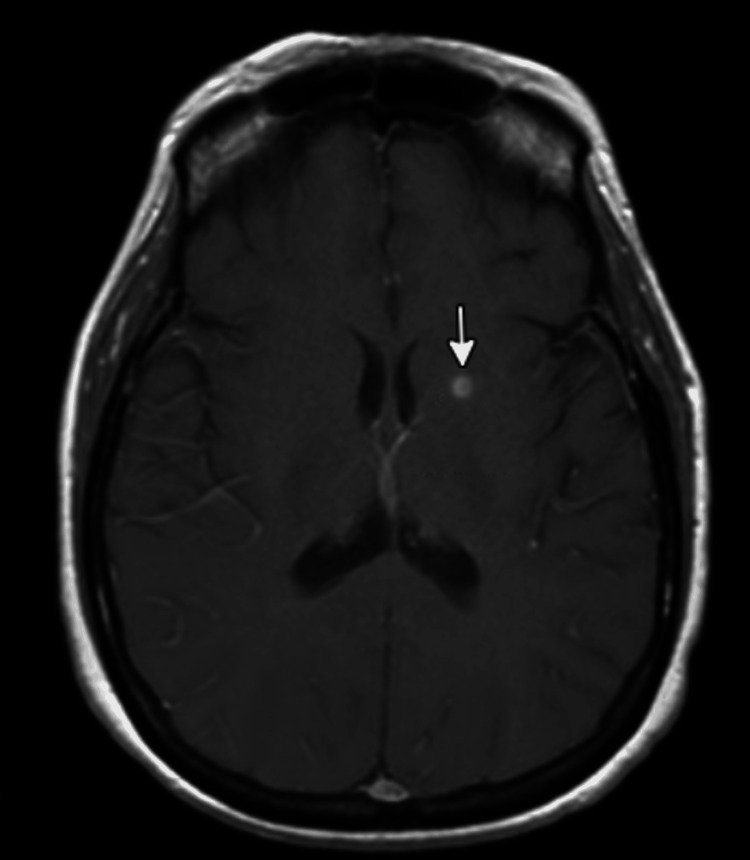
T1-weighted MRI with and without gadolinium contrast showing lesion concerning for infectious etiology. Ring-enhancing lesion within the left lentiform nuclei (white arrow).
